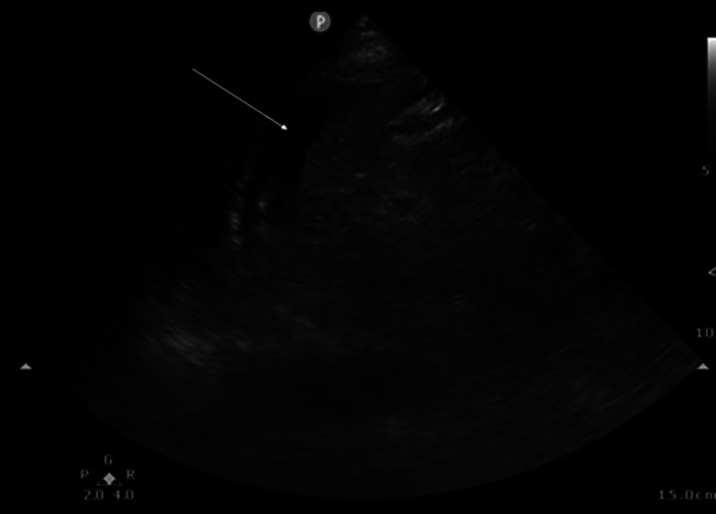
Point-of-care ultrasound showing free fluid in the left upper quadrant abdomen between the spleen and kidney (arrow).
